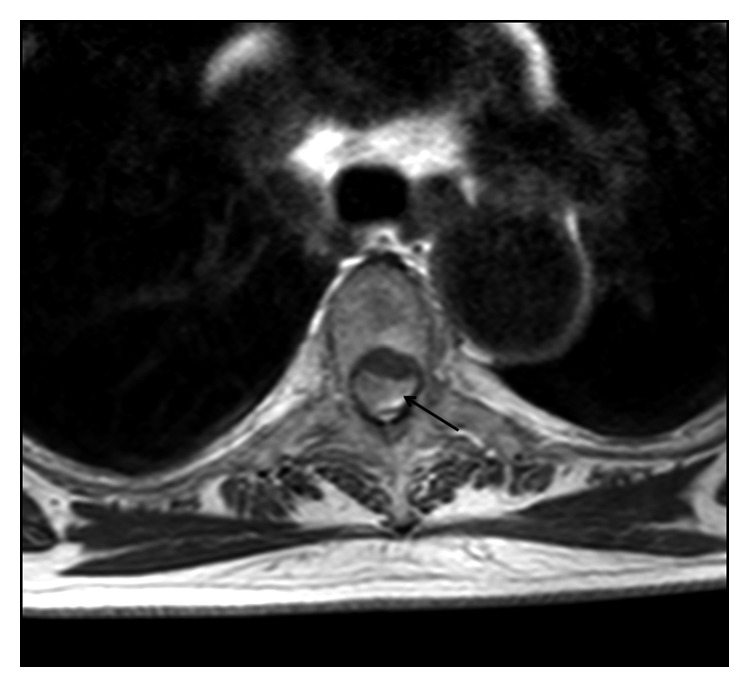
MRI scan: T1 transversal view of the dorsal column. Subacute subdural hematoma (→) with anterior displacement and compression of the spinal cord.
